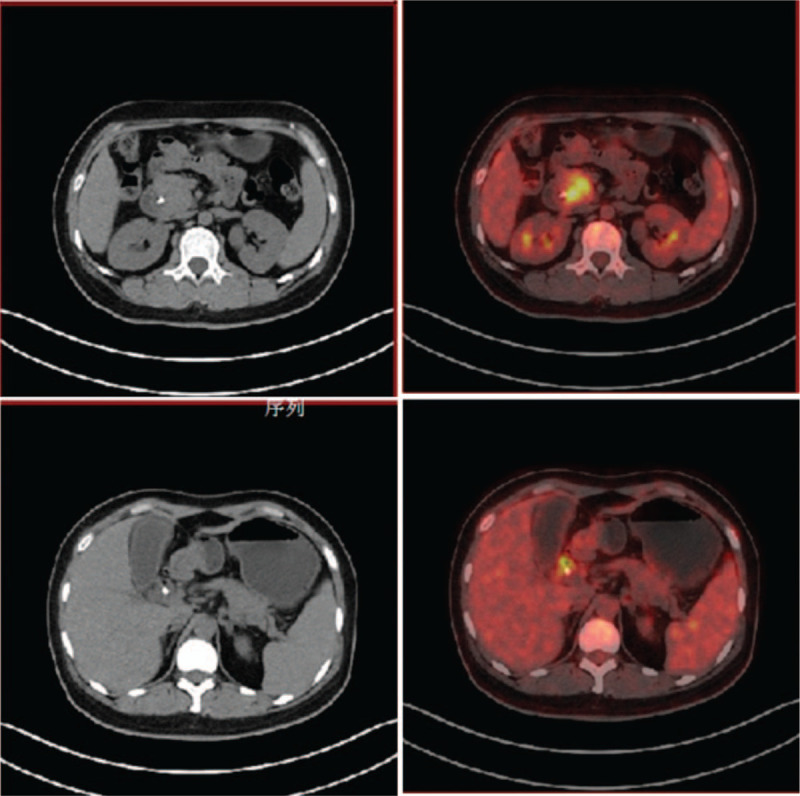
Soft tissue mass was seen in the head of the pancreas, the size was about 4.11 cm/3.79 cm, and the radioactivity uptake was increased. Interestingly, on PET-CT scan, liver lesions were invisible.
